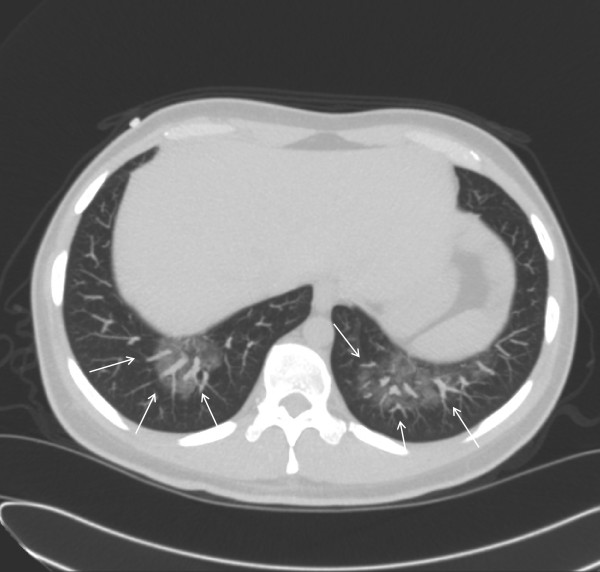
Chest-CT: Bilateral interstitial infiltrates.
